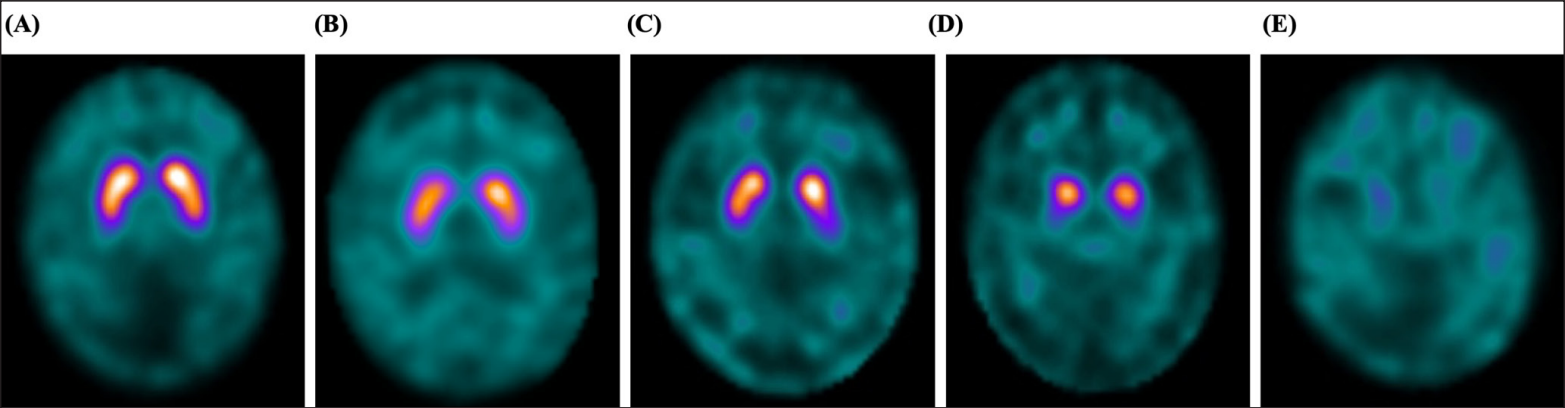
Kahraman’s visual assessment in ^123^I-FP-CIT SPECT images. (A) Grade 1 (normal), (B) Grade 2 (eagle wing), (C) Grade 3 (mixed), (D) Grade 4 (egg shape), and (E) Grade 5 (burst striatum).
